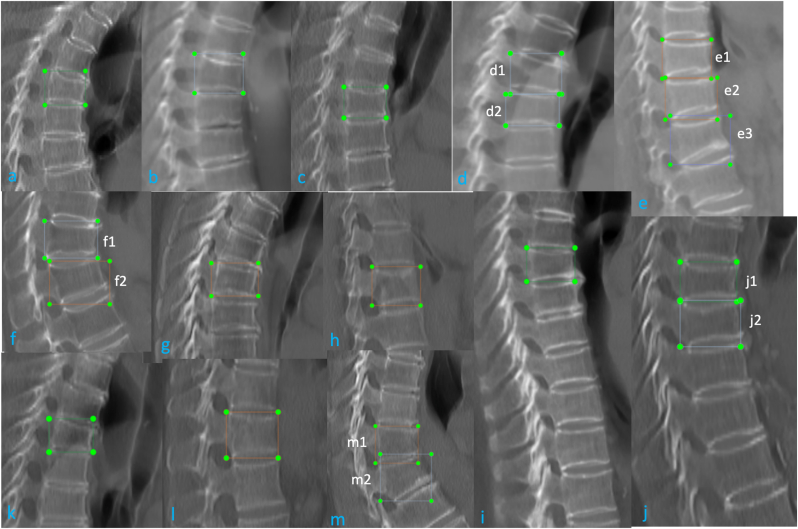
CSR CT images of 13 Cases with 19 VFs with false negative reading (labeled with green dotted box). Most of the missed VF are minimal or mild grades. In D, E, F, J, and M, missed VFs are associated with 2 or 3 adjacent VFs. Two adjacent VFs in D (d1 and d2); three adjacent VFs in E (e1, e2, and e3); two adjacent VFs in F (f1 and f2); two adjacent VFs in I (i1 and i2); and two adjacent VFs in M (m1 and m2).
